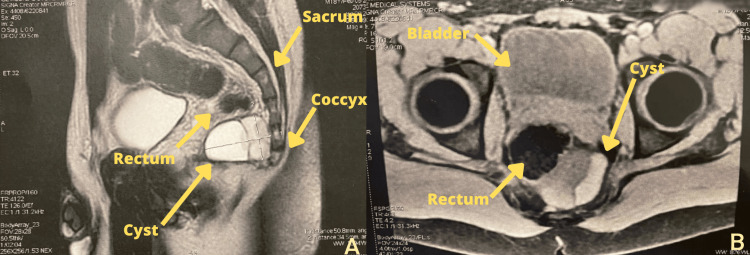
Cyst identification on magnetic resonance imaging (MRI) (A) Sagittal cross-section view; (B) axial cross-section view
